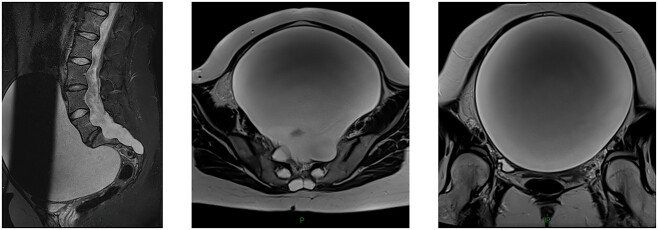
Lumbar magnetic resonance imaging in T2-weighted sequence showing an anterior sacral pseudomeningocele in a 27-year-old woman with Marfan syndrom. Left panel: sagittal view; Middle panel: axial view and Right panel: coronal view.
